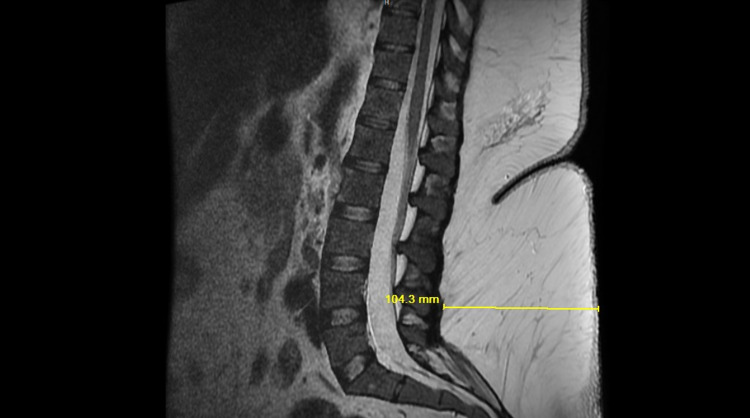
MRI Lumbosacral Spine MRI lumbosacral spine showing 104.3 mm depth of nerve roots through subcutaneous tissues from the skin.
